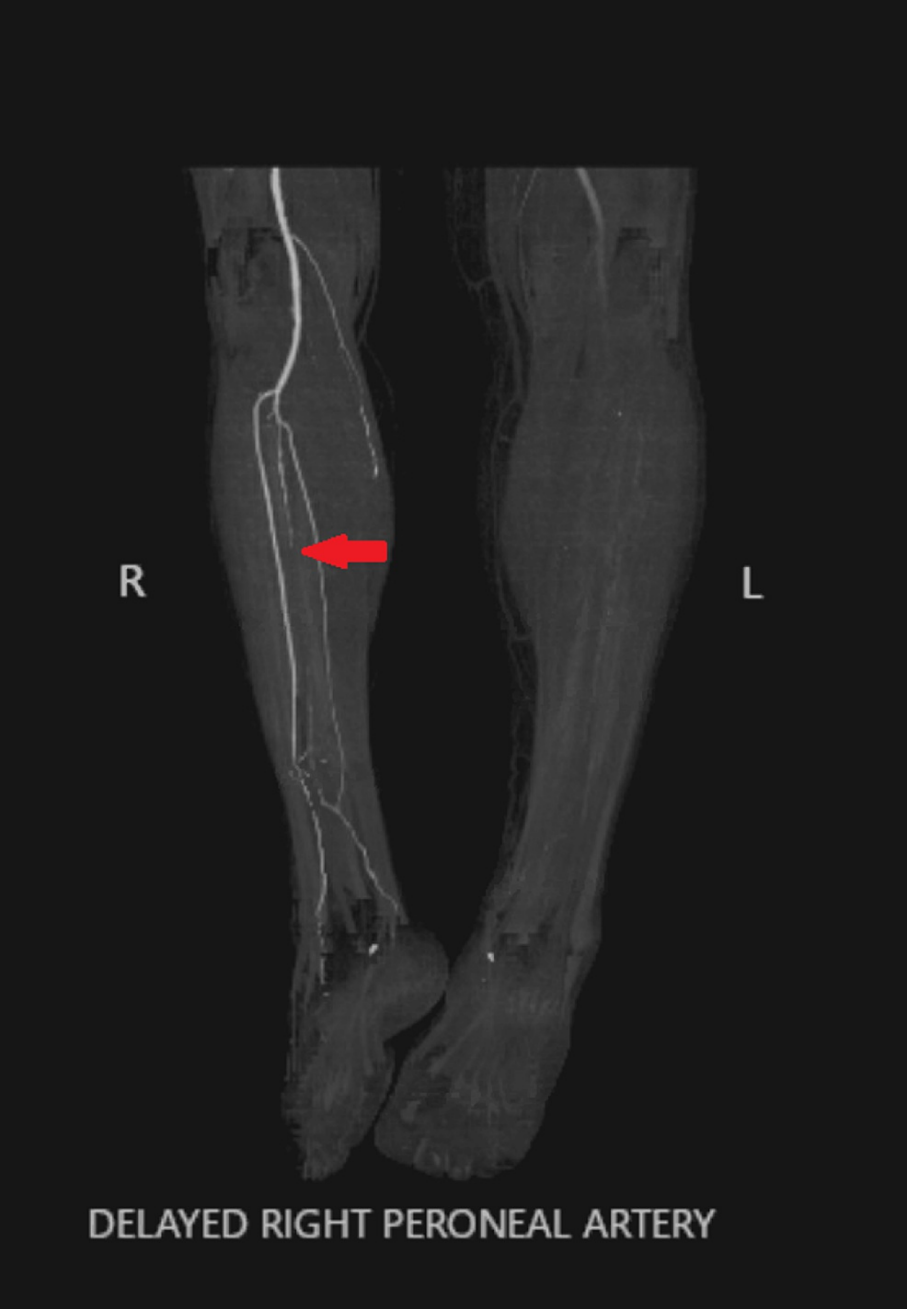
CT angiography imaging demonstrating occlusion of the right peroneal artery in the mid leg at the level of the arrow
